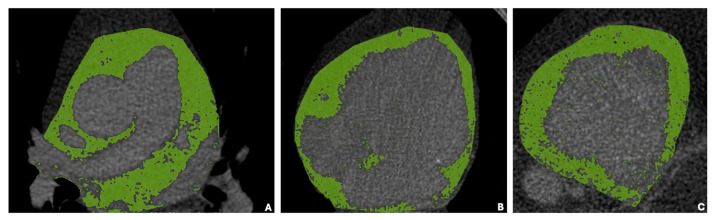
(A–C) Non-contrast cardiac CT axial views at three different anatomical levels of the same patients (EAT volume: 414 cm^3^). (A) shows pericardial contours drawn at the most superior slice, at the bifurcation of the main pulmonary artery; (B) pericardial contours at the medial slice and (C) at the most inferior slice, where the posterior descending coronary artery in the inferior atrioventricular groove is last seen. The segmentation process of the epicardial adipose tissue (green areas) was completed using the quantification software.
